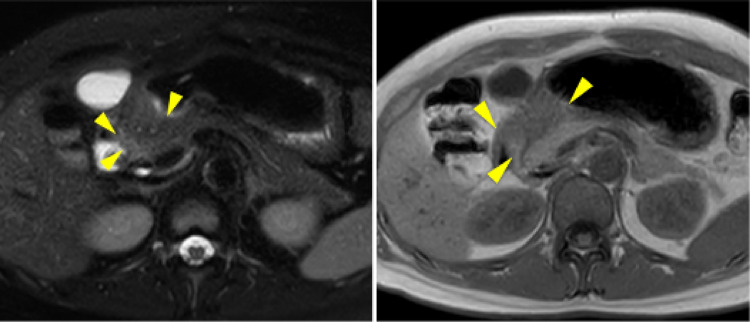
Magnetic resonance imaging (T2-weighted fat-suppressed and T1-weighted sequences) showing tubular structures broadly contacting the pancreatic parenchyma
